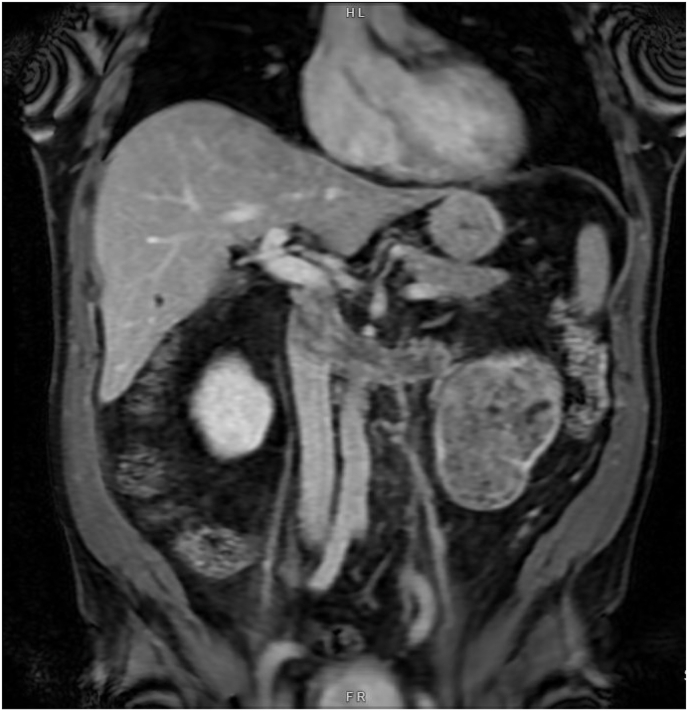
MRI of the abdomen and pelvis showing left renal mass with tumor thrombus occupying the renal vein and IVC.
